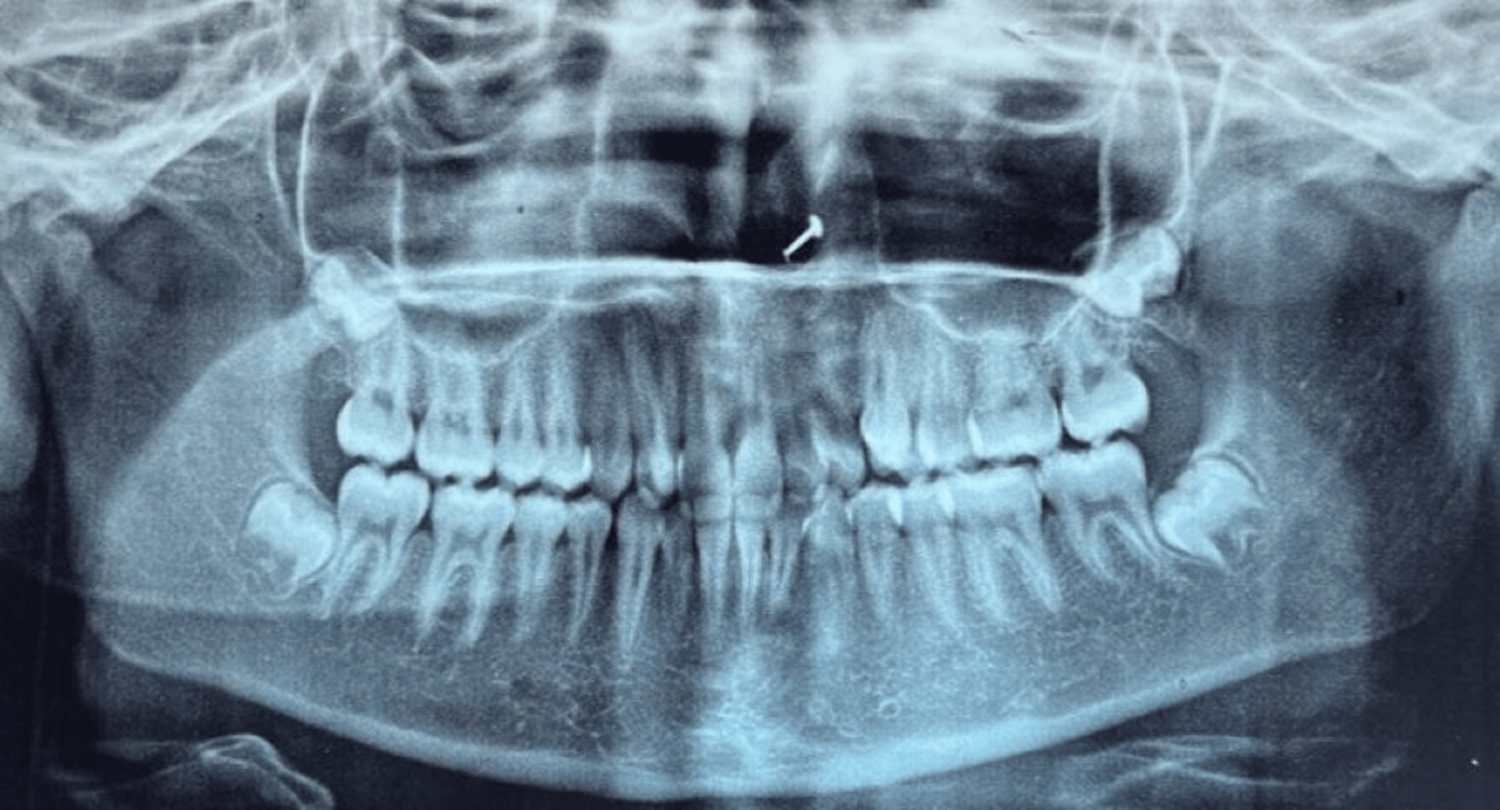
Orthopantomogram showing complete root development.
